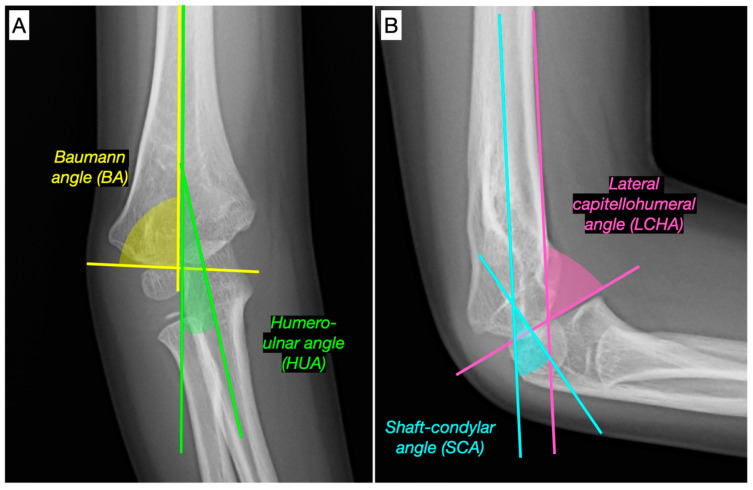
Radiologic measurements on anteroposterior (A) and lateral (B) elbow radiographs. BA, Baumann angle; HUA, humero-ulnar angle; SCA, shaft-condylar angle; LCHA, lateral capitellohumeral angle.
